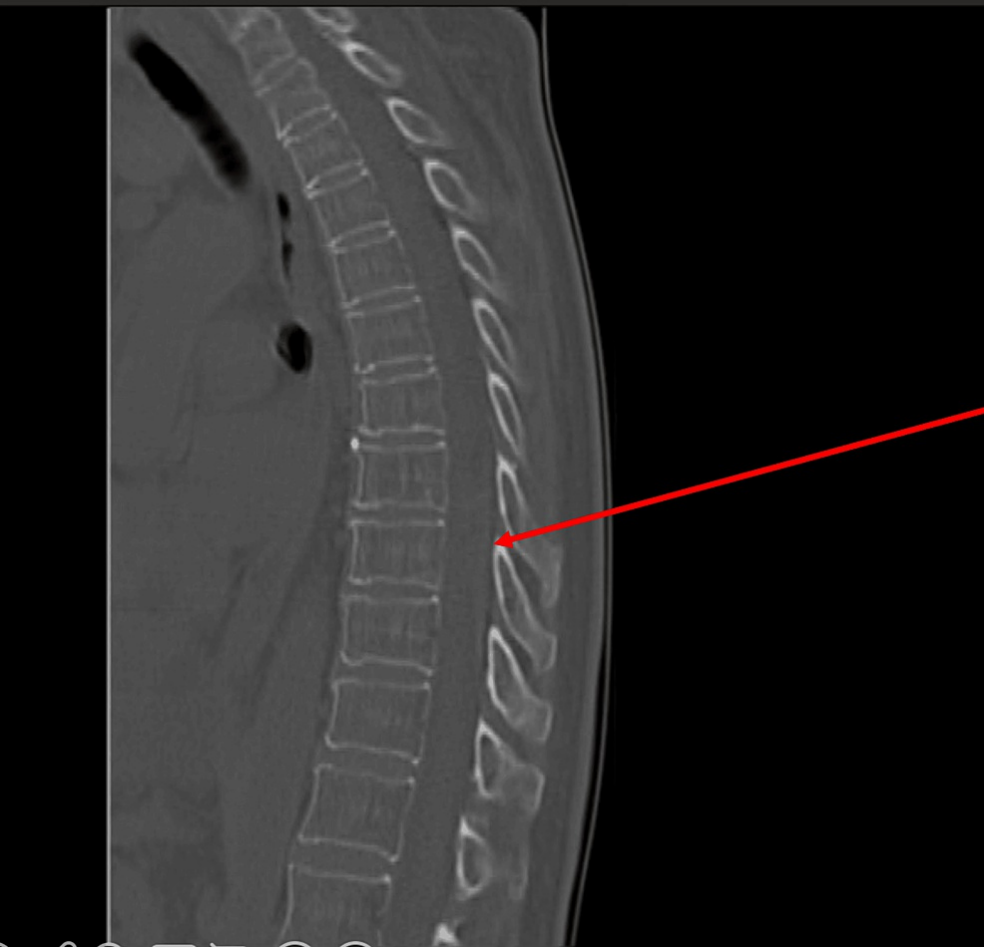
Thoracic and lumbar CT scan A hyperintense lesion displacing the cord anteriorly at T8-T10 (indicated by red arrow).
